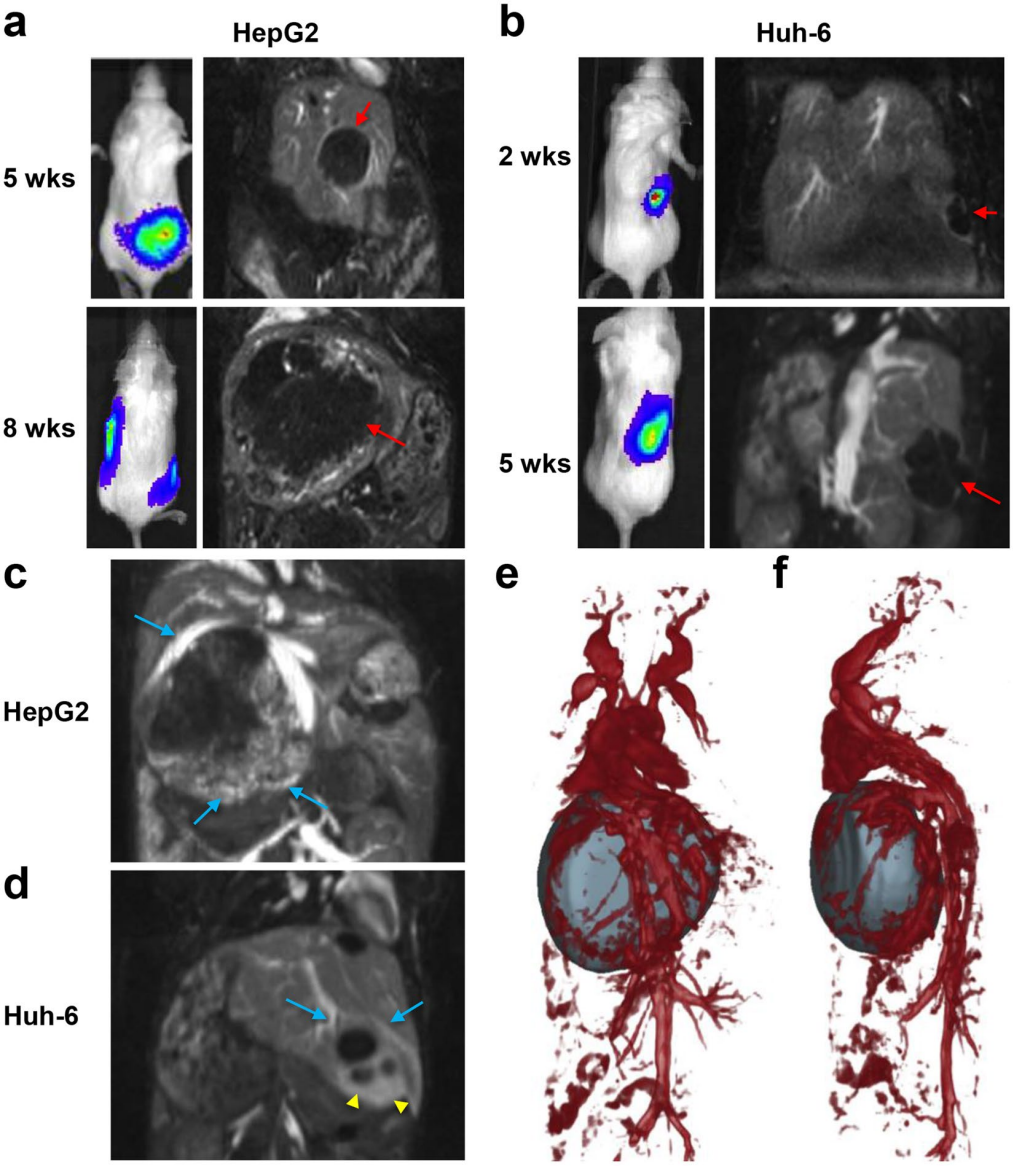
MRI of xenograft tumors and tumor-associated vasculature. (a,b) Representative contrast-enhanced T1-weighted coronal images showing the presence of tumor masses (red arrows) at early and late time points. Representative BLI images acquired on the same day are shown for comparison. (c,d) Representative contrast-enhanced T1-weighted coronal thick slab maximum intensity projection (MIP) abdominal images demonstrating the presence of increased vascularity (blue arrows) and highly permeable vessels (yellow arrowheads) in the peripheral regions of the tumors. (e,f) Coronal (e) and sagittal (f) 3D volume rendering MRI of HepG2 tumor and major hepatic blood vessels.
